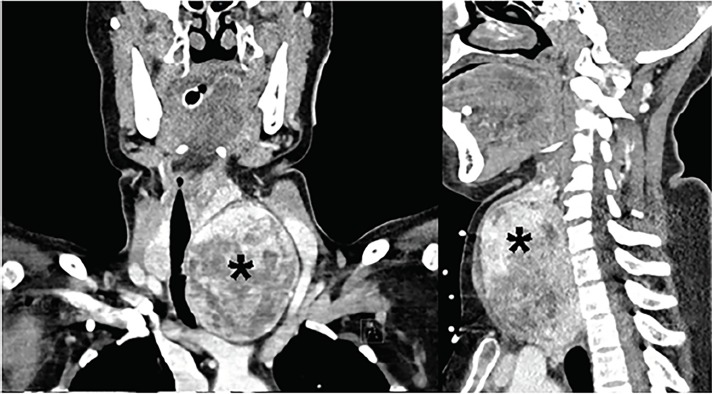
Computed tomography of neck and soft tissues showing a large substernal goiter (*) with tracheal compression (frontal plane on left, sagittal plane on right).
